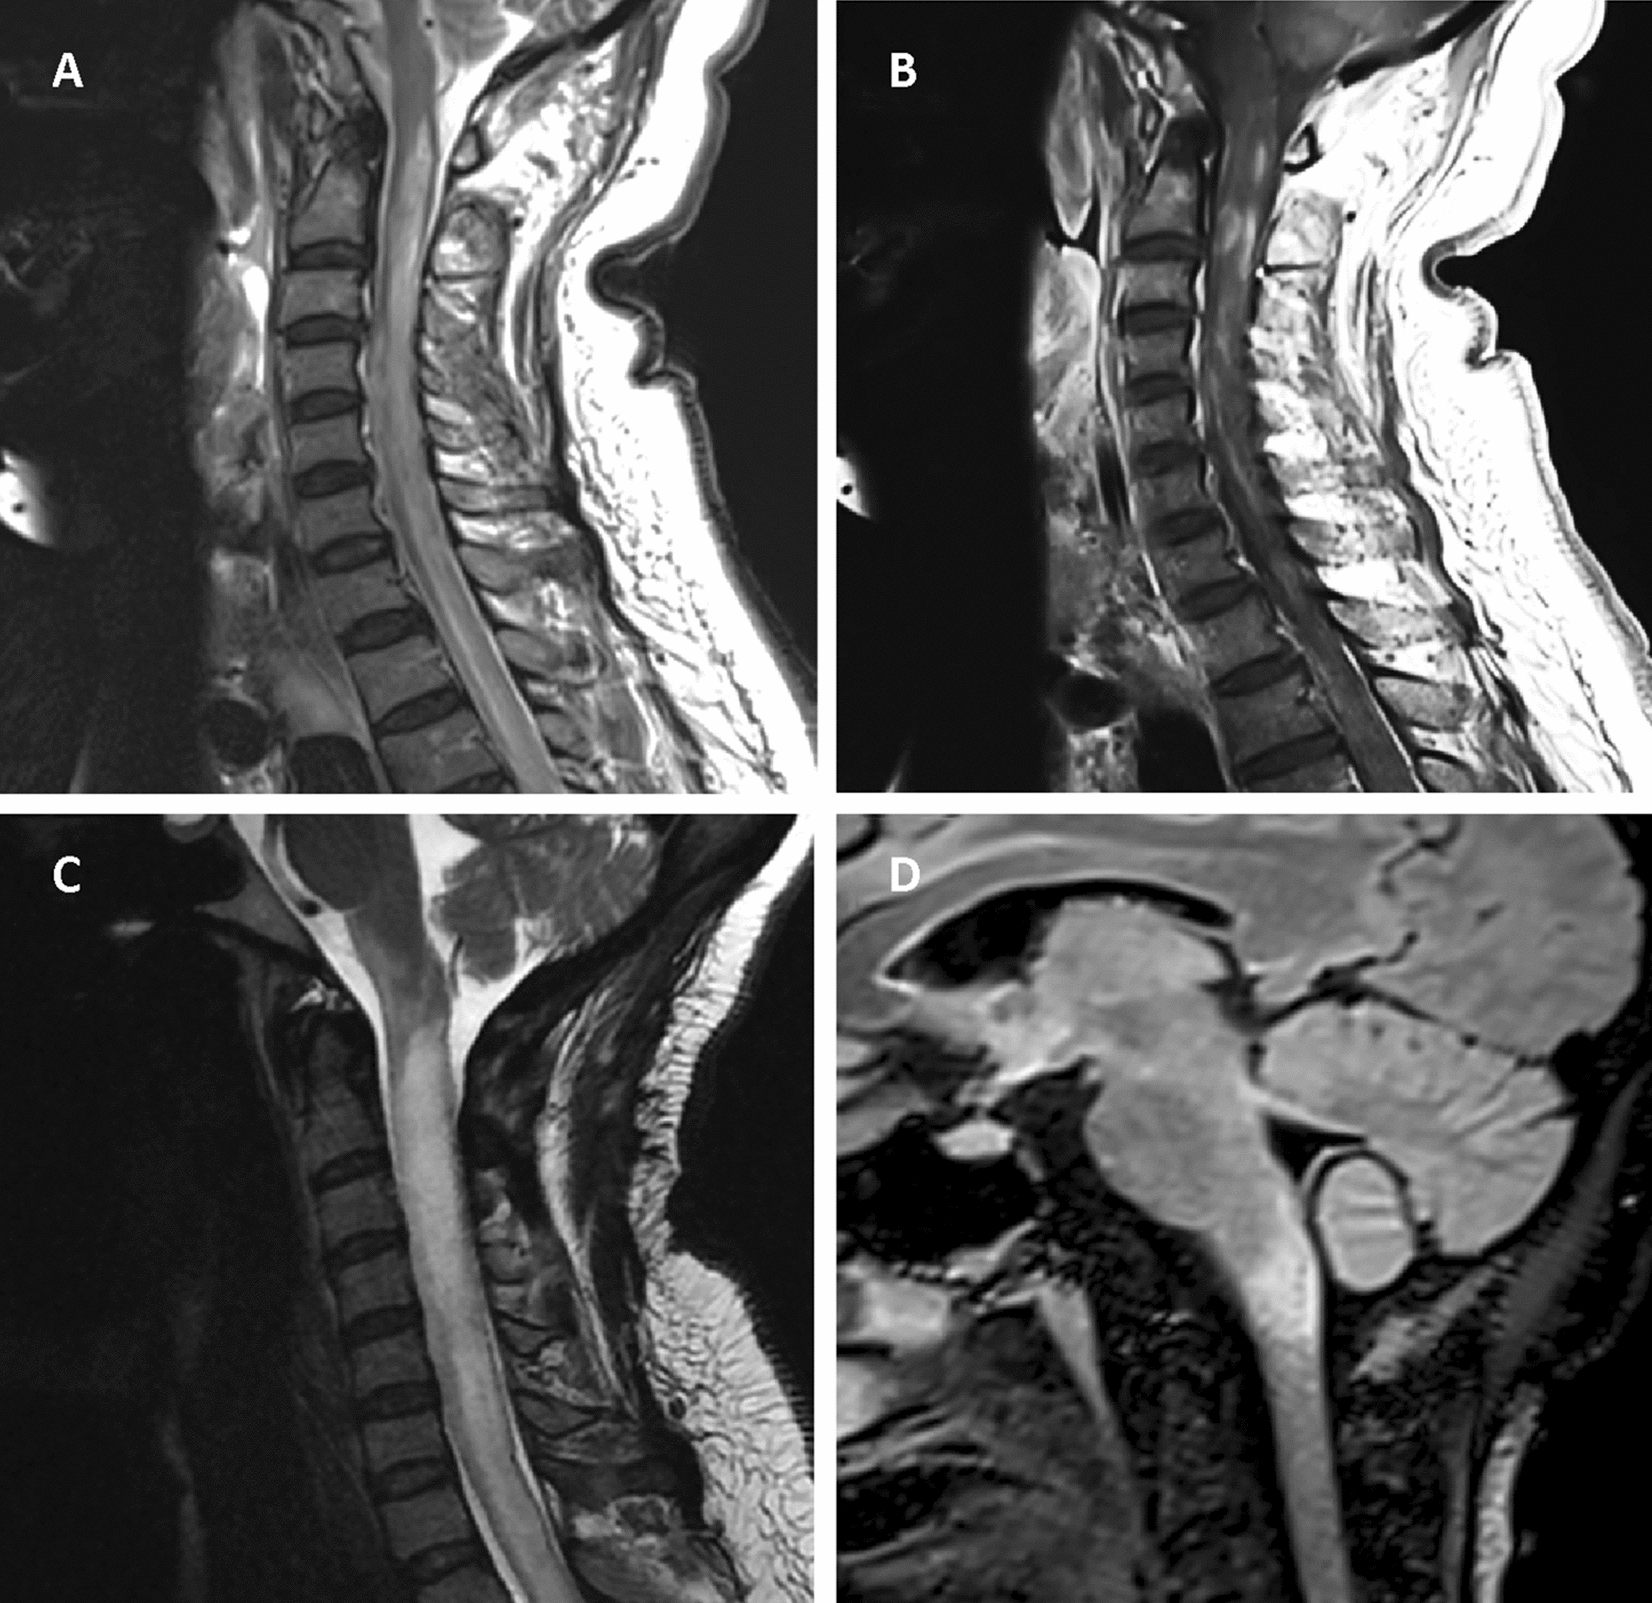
MRI features of NMOSD-AQP4+ patients with OTI-attacks. A, B Are derived from the same patient. A, C Are sagittal spinal cord T2 MRI. B Is sagittal T1 with gadolinium. D Is FLAIR MRI
